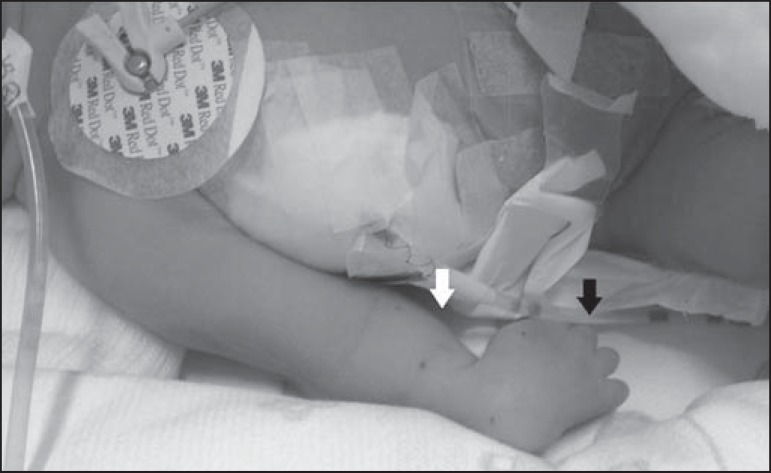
Ultrasound image of the newborn, showing the shortening of the forearm (white
